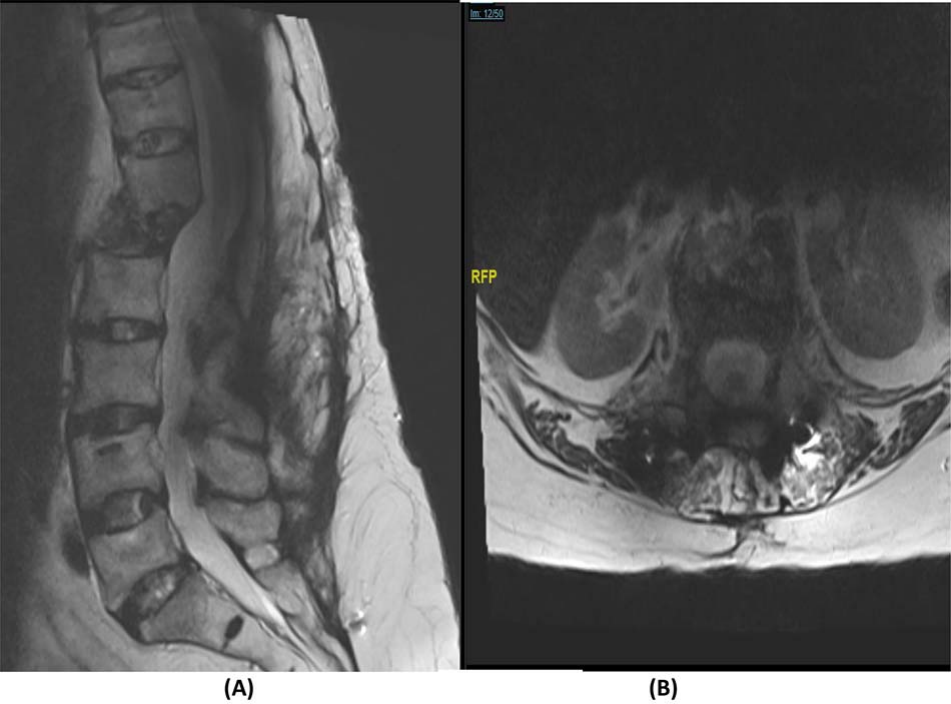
(A) T2-weighted sagittal spine MRI demonstrating changes at the thoracolumbar spine with complete resolution of infection and no cord compression. (B) T2-weighted axial spine MRI of the corresponding level of L1 vertebral body.
